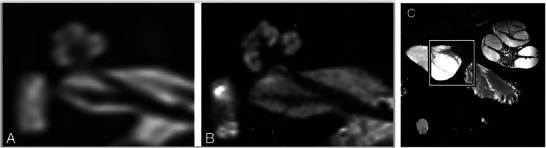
3T (A), 7T (B), and 11.7T MRI of the human cochlea. Axial cross‐section of a human inner ear and cochlea in vivo using 3T (A) and 7T (B) MRI. Reproduced from van der Jagt et al. with permission.^[^
